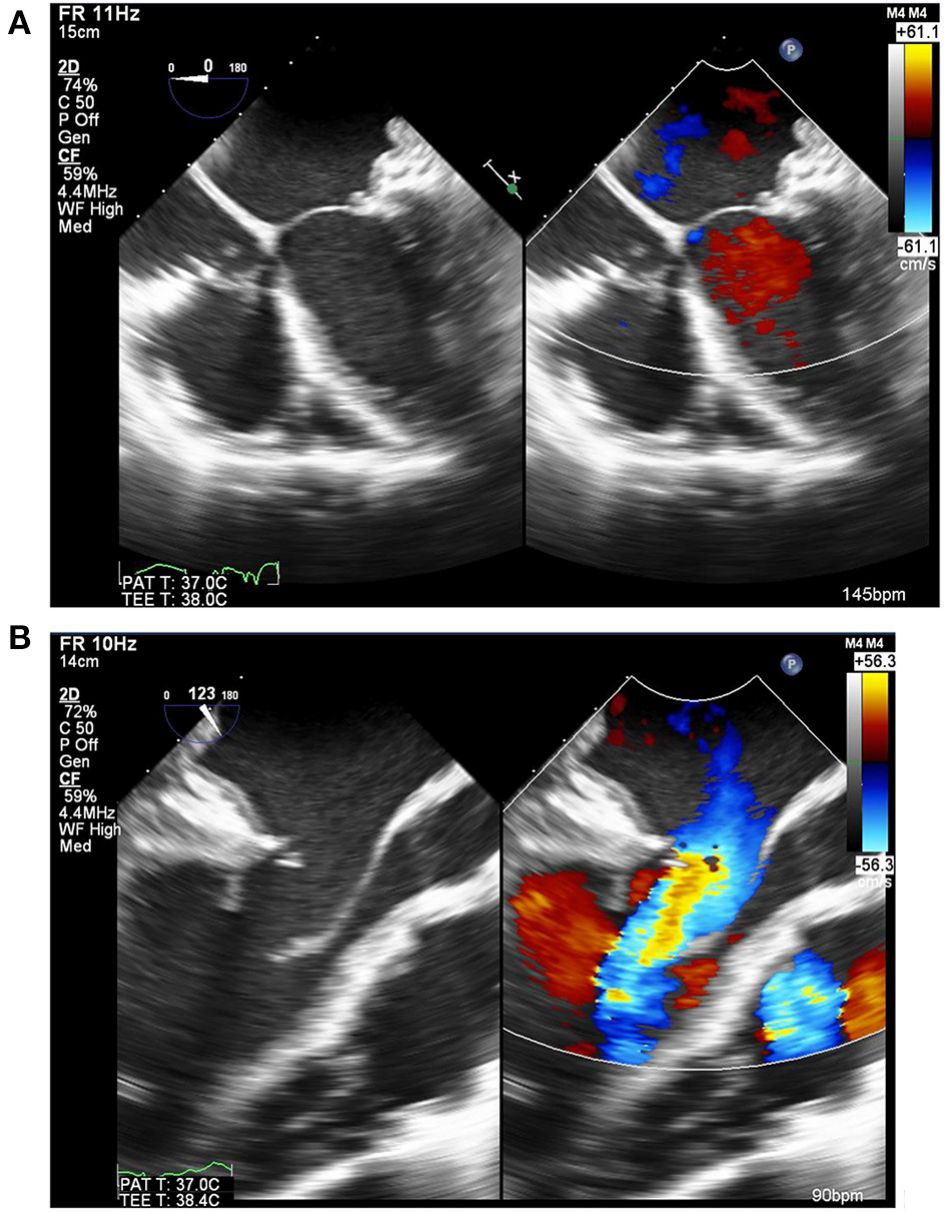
Echocardiogram of the mitral valve after repair by peeling of the anterior leaflet coupled with a pericardial posterior annuloplasty. This showed good coaptation (A), laminar flow through the valve and excellent mobility of the leaflet (B) [Courtesy of Aswan Heart Center (AHC) database].
